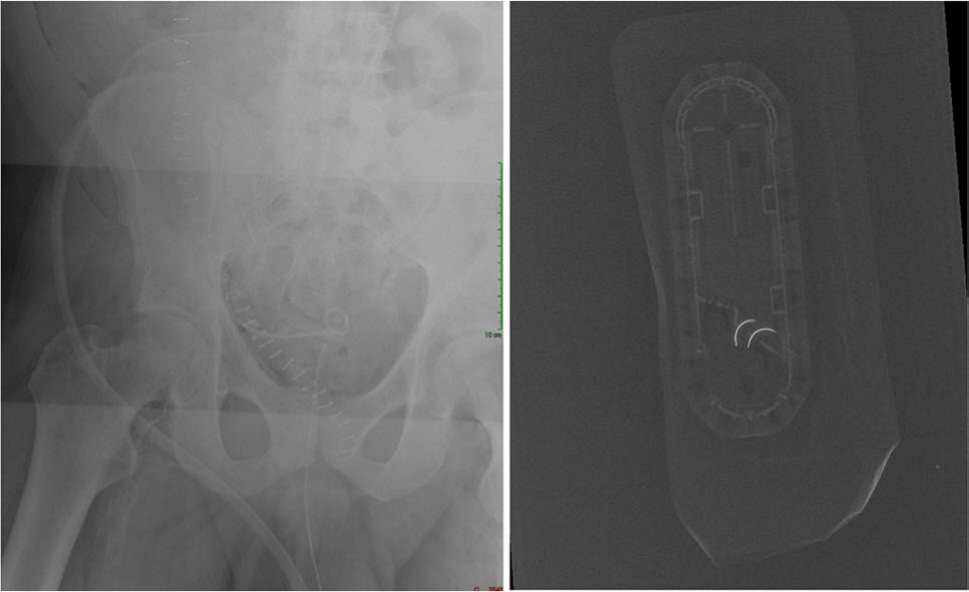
72-year-old male undergoing renal transplantation. Due to an incorrect count, an intraoperative X-ray was performed (A), which was negative for any retained metallic device. The optimal protocol in these clinical scenarios involves providing the interpreting radiologist an image of the missing foreign body (B).
